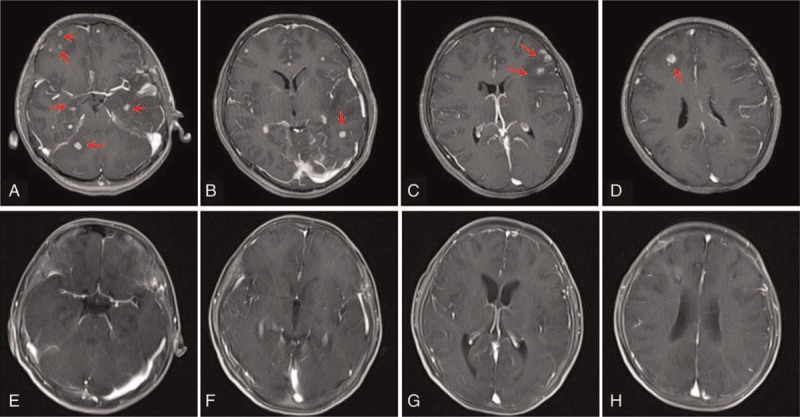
Pre- and postoperative contrast MRI of Case 4: (A–D) preoperation, multiple intracranial lesions (right cerebellum, right and left frontal lobe, left temporal lobe) could be found, as indicated by the red arrows. (E–H) 3 months postoperation, after the suitable treatments, the multiple intracranial lesions disappeared. MRI = magnetic resonance imaging.
